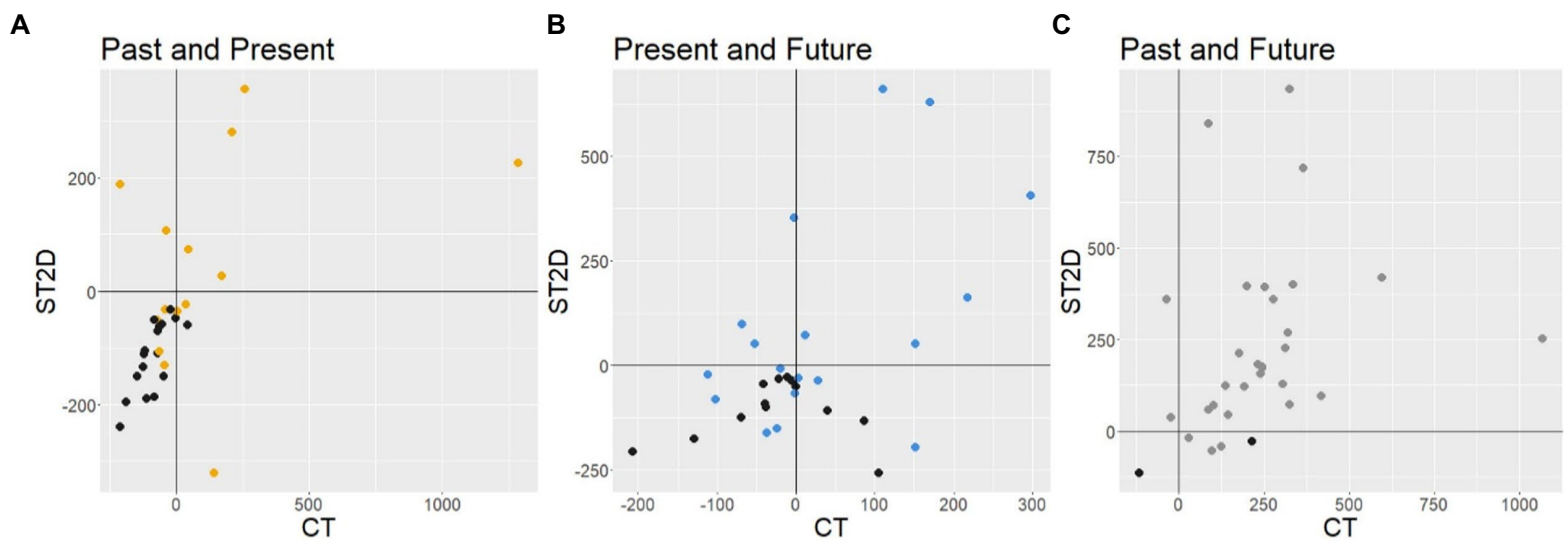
Distance between the surfaces of spheres in ST as a function of the distance between surfaces of circles in CT. Each dot expresses the past, present, and future positions of a participant. Black dots indicate that the two spheres are attached to each other in the 3D space in ST. (A) Past-Present distance on the screen in ST. (B) Present-Future distance on the screen in ST. (C) Past-Future distance on the screen in ST.
